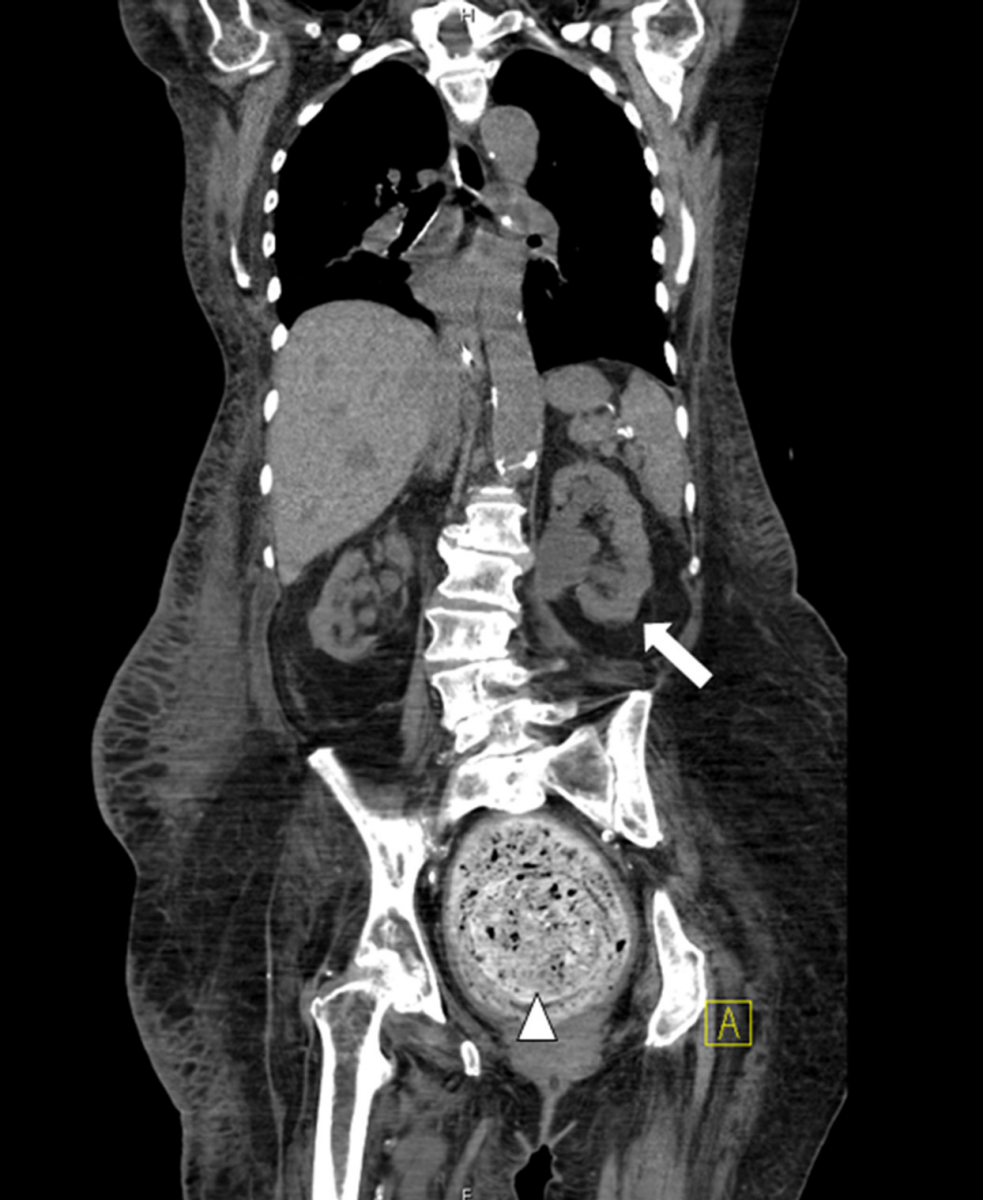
Noncontrast CT abdomen/pelvis, coronal view. Rectal fecalith (14 cm; triangular arrow) with subsequent compression of the ureters and resulting bilateral hydronephrosis and hydroureter (white arrow).
